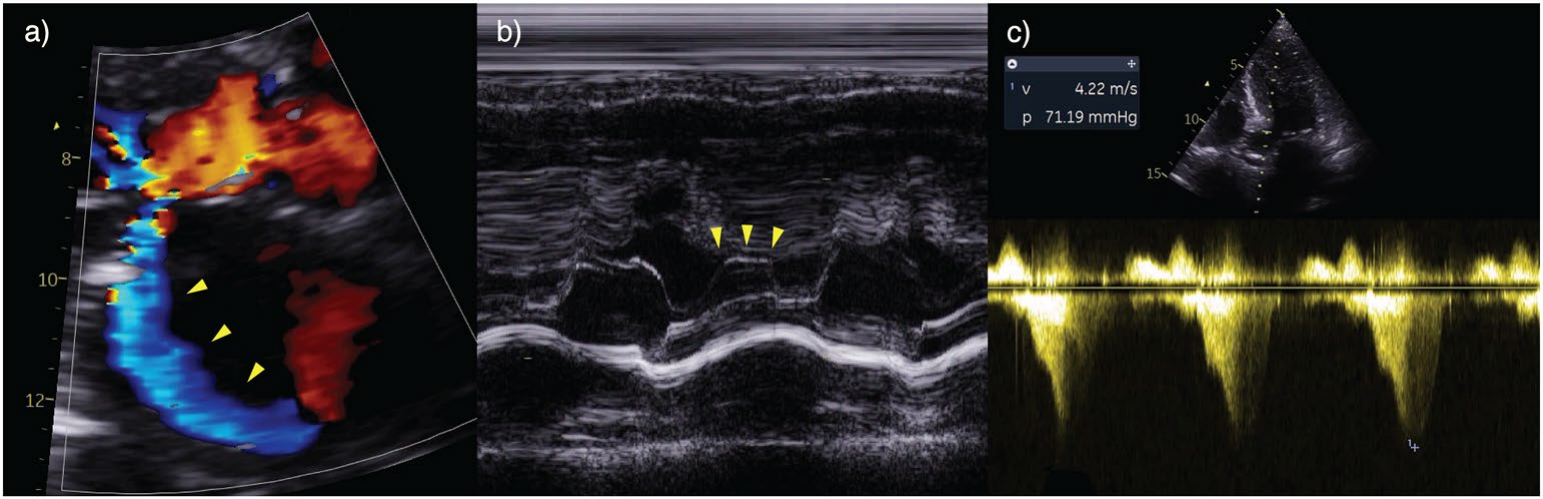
Case 2—LVOT obstruction. a Posteriorly directed MR jet (arrows) in the parasternal long-axis view; b M-mode demonstrating systolic anterior motion of the mitral valve (arrows); c spectral Doppler showing dynamic LVOT obstruction with a peak gradient of 71 mmHg
